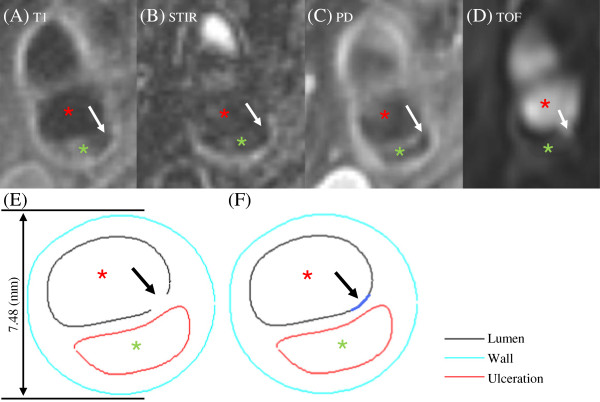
High resolution, multi-sequence MR image and corresponding segmentation contour. (A-D): MR images showing fibrous cap rupture (marked by white arrow) and ulceration; (E): segmented contour with a defect lumen due to fibrous cap rupture; and (F): cubic spline function was used to bridge the gap (thick blue line) to recover the intact lumen. Red asterisk: lumen; Green asterisk: ulceration.
